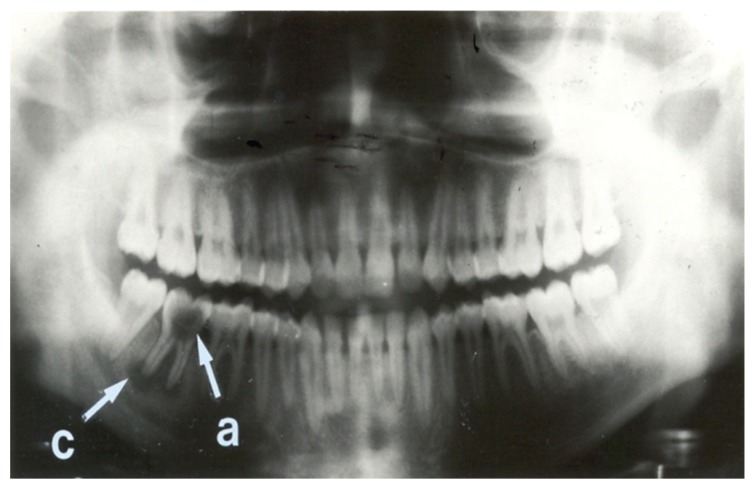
Panoramic radiograph showing few abnormalities. This radiograph shows complete dentition, with no unerupted or impacted teeth. Interdental bone loss is minimal. A large carious lesion (a) is present in the right second mandibular molar tooth. A peri-apical radiolucency (c) is also present at the apex of the same tooth. This is indicative of a peri-apical abscess or granuloma.^21^ The overall condition of the teeth of this patient is good.
